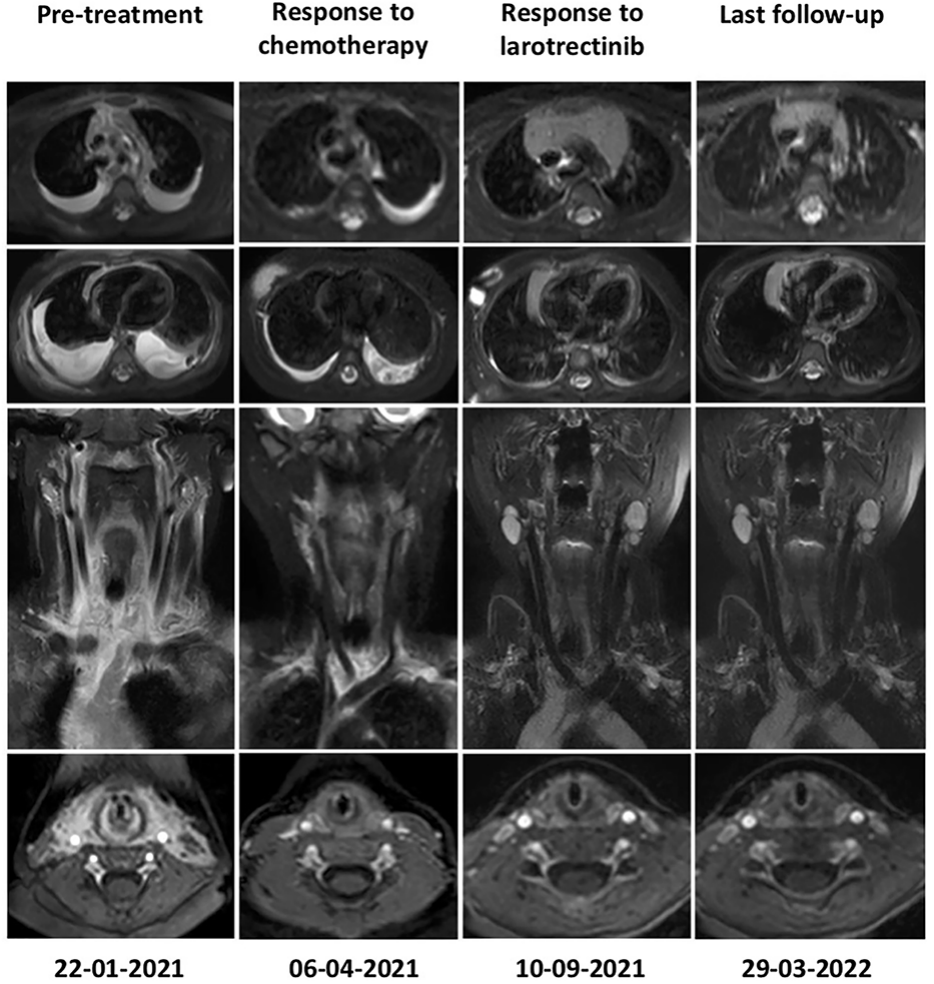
Radiological response as determined by Magnetic Resonance imaging before treatment (22.01.21), following administration of the CWS 2012 protocol (06.04.21) and NTRK inhibitor therapy (Larotrectinib) (10.09.21), respectively. Follow-up MRI is performed every three months; the latest images show a sustained response to Larotrectinib therapy as no visible tumour mass is detectable (29.03.22). While a limited therapeutic response upon completion of chemotherapy, a remarkable and sustained complete remission from Larotrectinib was observed.
